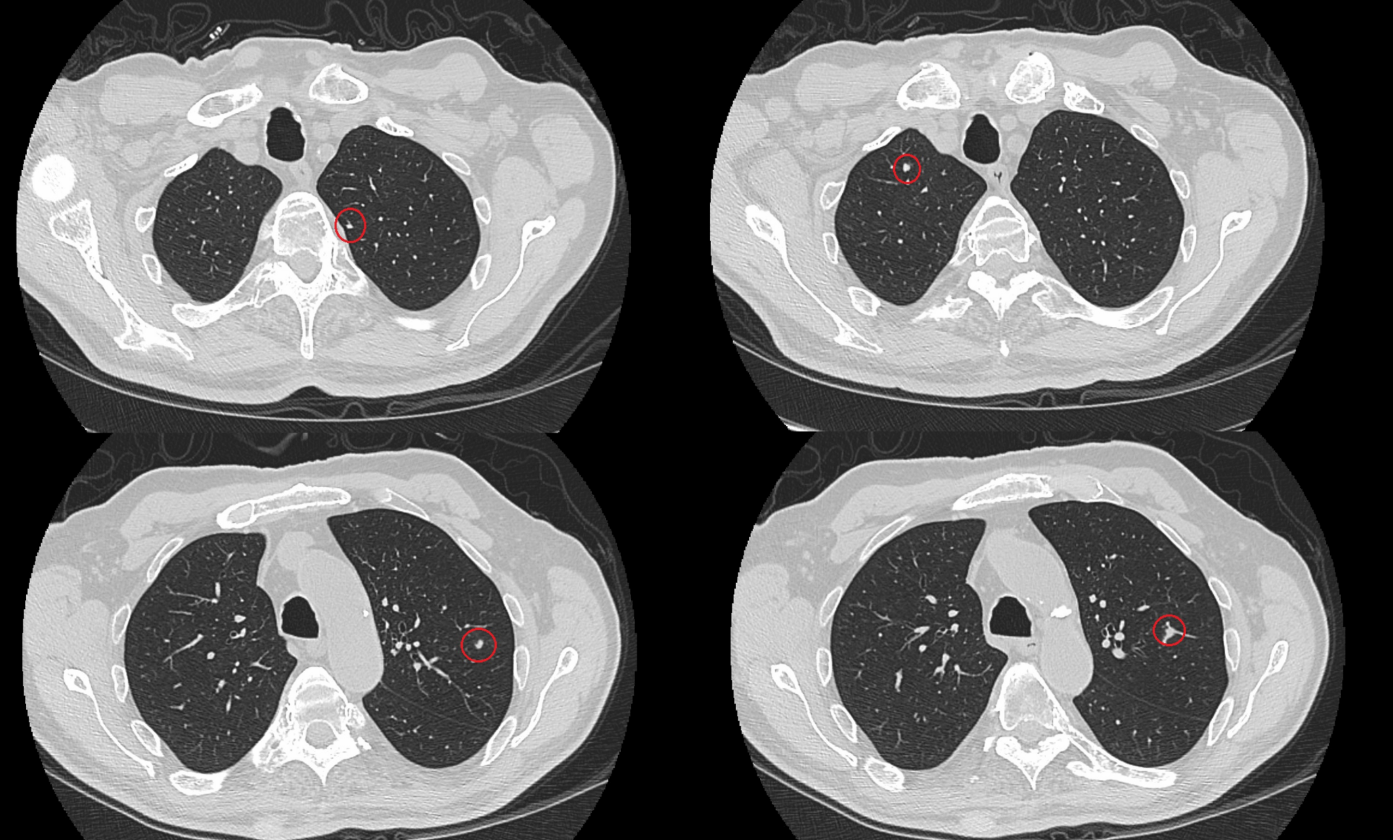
Axial chest CT scan showing pulmonary nodules highlighted in red
